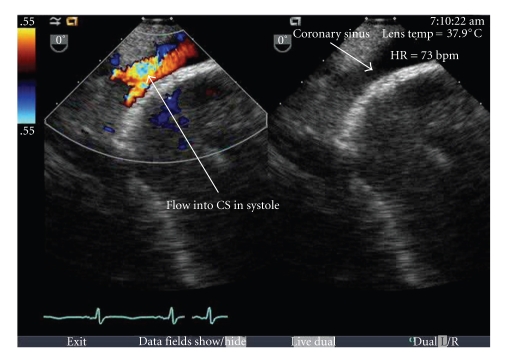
TEE showing doppler signal away from LA. The arrows indicate a dilated coronary sinus.
